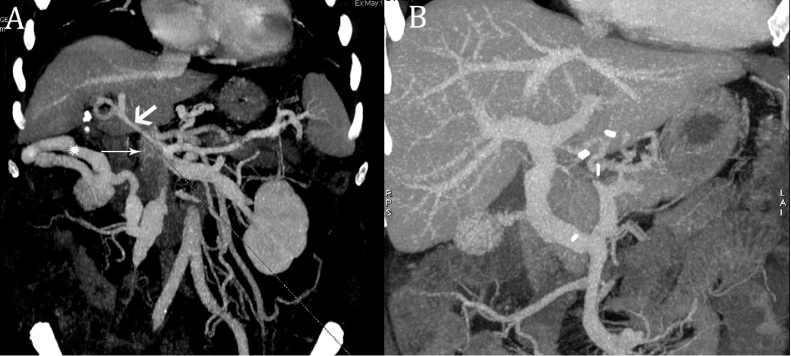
Multiplanar contrast-enhanced computed tomography (CT) 3D reconstructions. A: pretransplant CT showing grade 3 portal vein thrombosis, with proximal involvement of the superior mesenteric vein (thin arrow). A thin portal vein collateral is identified at the hepatoduodenal ligament (thick arrow), as well as a large portosystemic shunt (asterisk) arising from distal superior mesenteric vein and draining into the inferior vena cava, below the outlet of right renal vein. B: post-transplant CT showing patency and position of the mesoportal jump graft.
